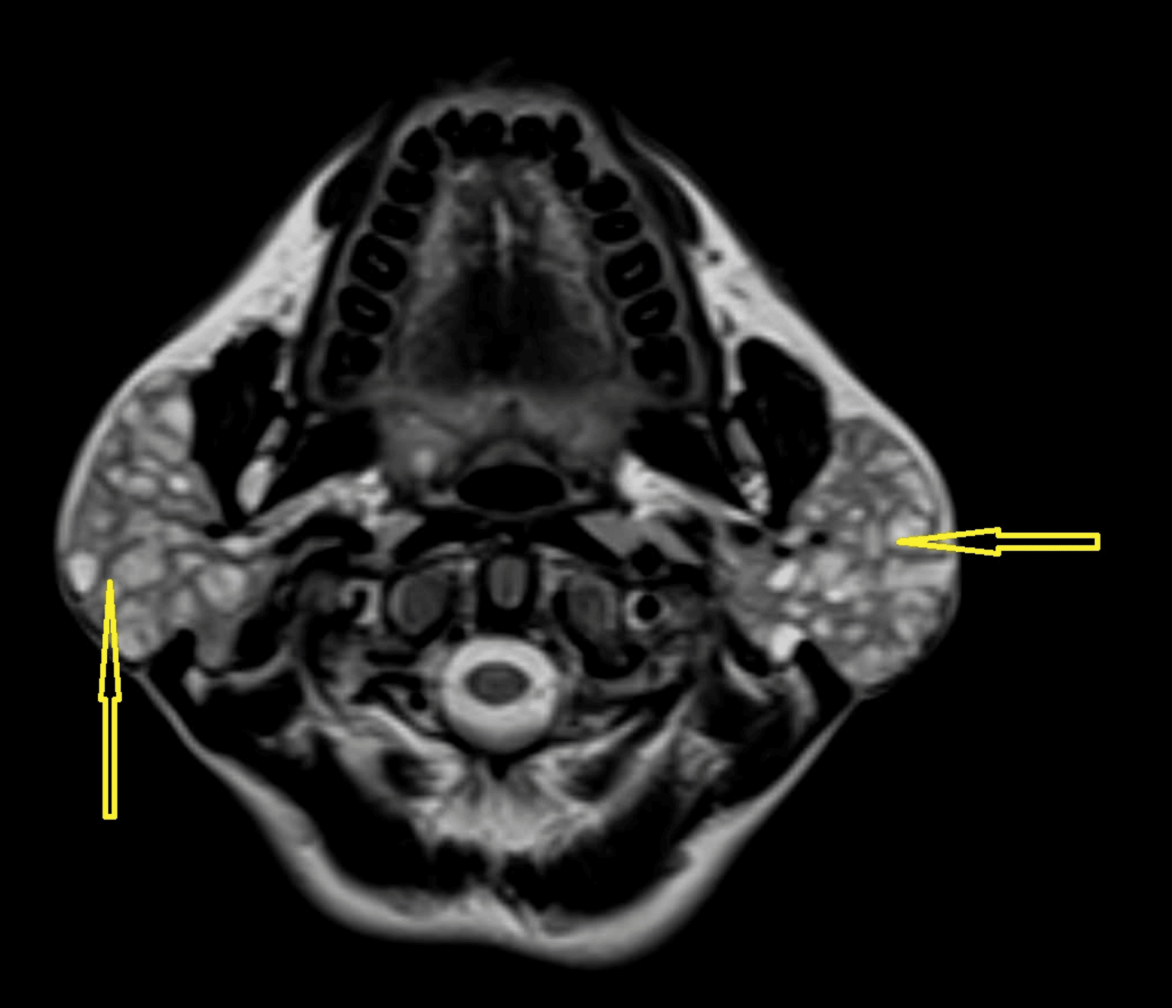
T2-weighted axial image showing the hyperintense lesions in the bilateral enlarged parotid glands
